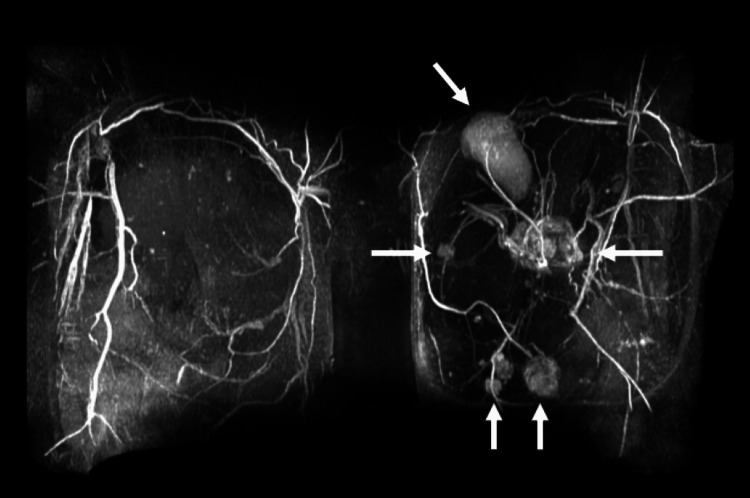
3D-reconstruct of MRI breast showing multifocal left-sided breast lesions (antero-posterior view). MRI: magnetic resonance imaging
